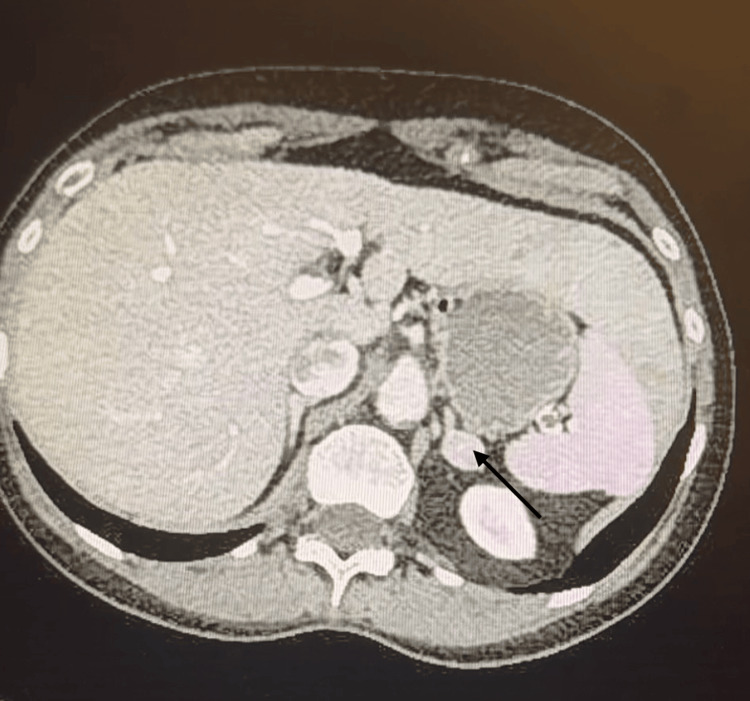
Abdominal CT revealing the adrenal mass (black arrow)
